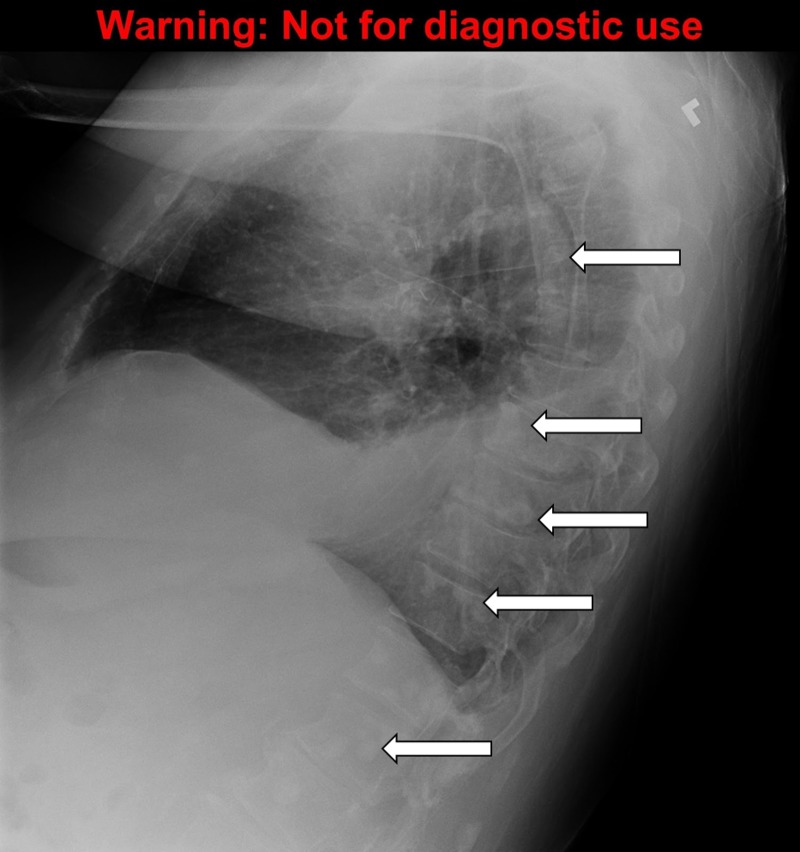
The chest X-ray, lateral view demonstrating bone metastasis. Source: A Dixit
